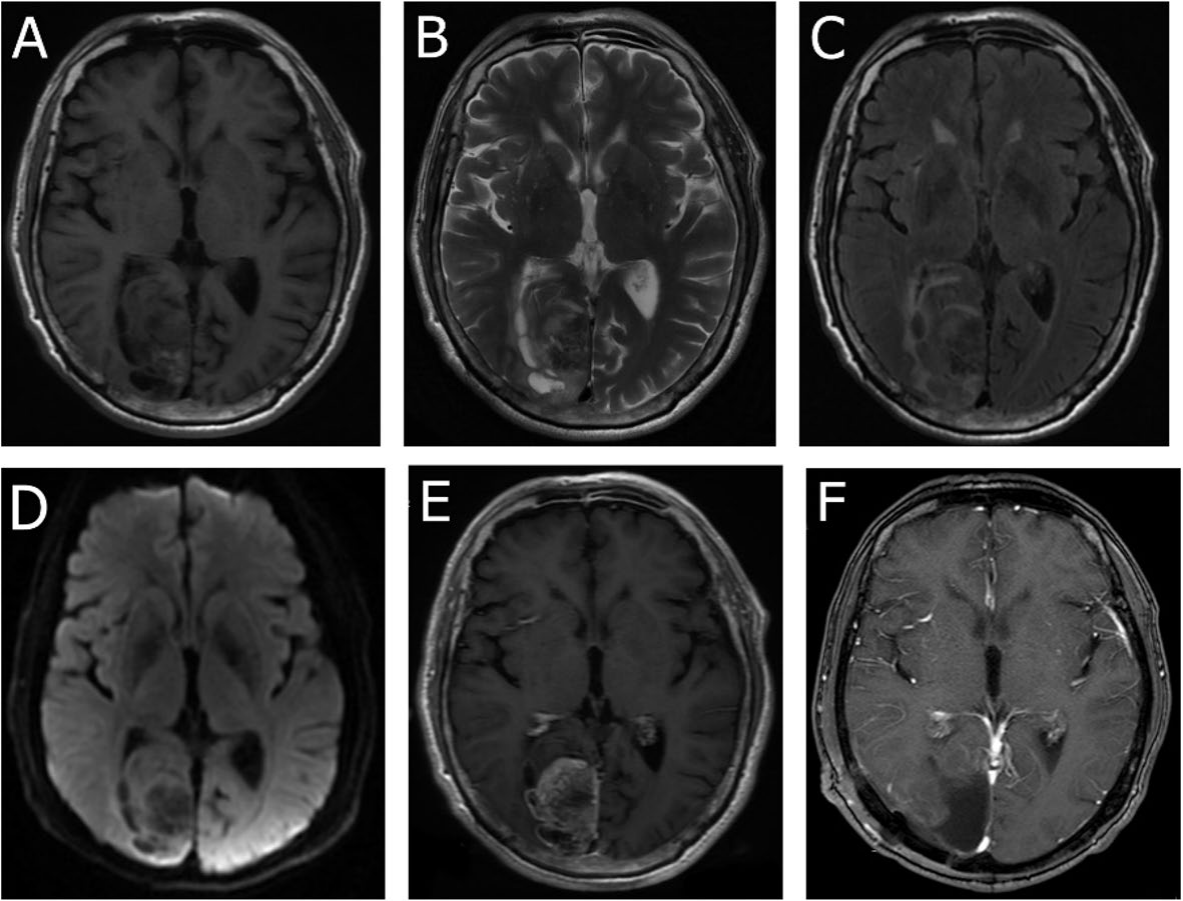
Preoperative MRI showing a mass in the right occipital lobe. A T1w image showing low and high mixed signals. B-C T2w and T2 FLAIR images showing high and slightly low mixed signals. D DWI showing a low signal in the lesion. E Image showing uneven enhancement. F Postoperative MRI confirmed that the tumor had been completely removed
